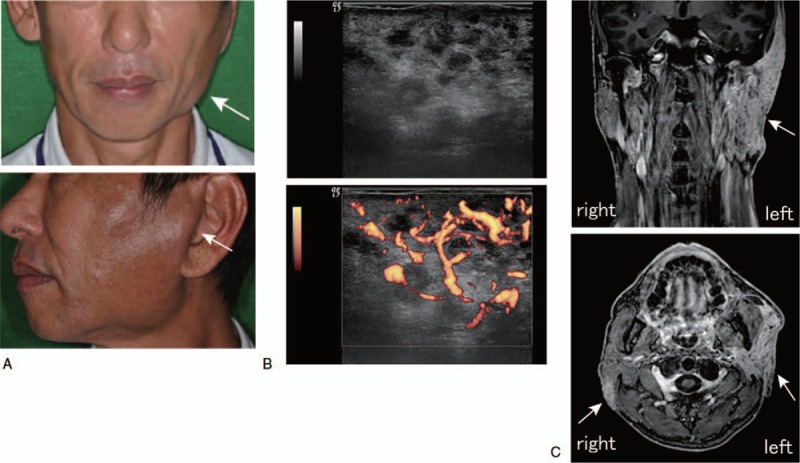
(A) Kimura disease: the patient presented with swelling of left parotid gland. (B) (Upper) Transverse US of the left parotid gland revealed a reticular pattern. (Lower) Power Doppler US revealed enriched vascularity in the gland. (C) MRI T1 weighted image with gadolinium enhancement showed multiple mass lesions (white arrow) involving the swollen left parotid gland and the swollen subcutaneous soft tissues. MRI = magnetic resonance imaging.
